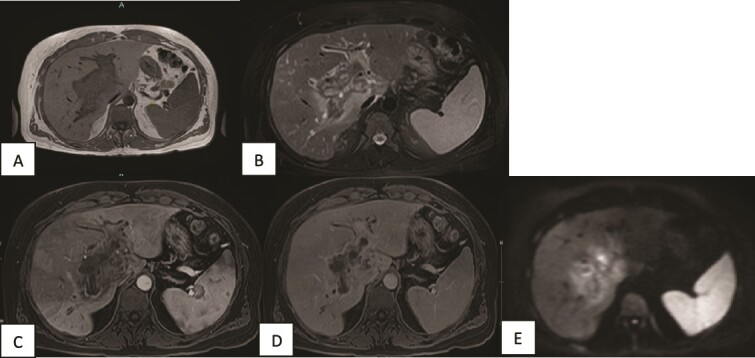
MRI of the liver (A, B) Axial T1WI and T2WI fat-saturated images revealing an isointense central rim with hypointense periportal edema on T1-weighted images and a hypointense rim along the portal tracts with periportal edema on T2-weighted images. (C, D) Axial T1WI postcontrast images obtained at the arterial and porto-venous phases showing mild peripheral enhancement with non-enhancement of the portal vein. (E) DWI showing minimal diffusion restriction at the hilum.
